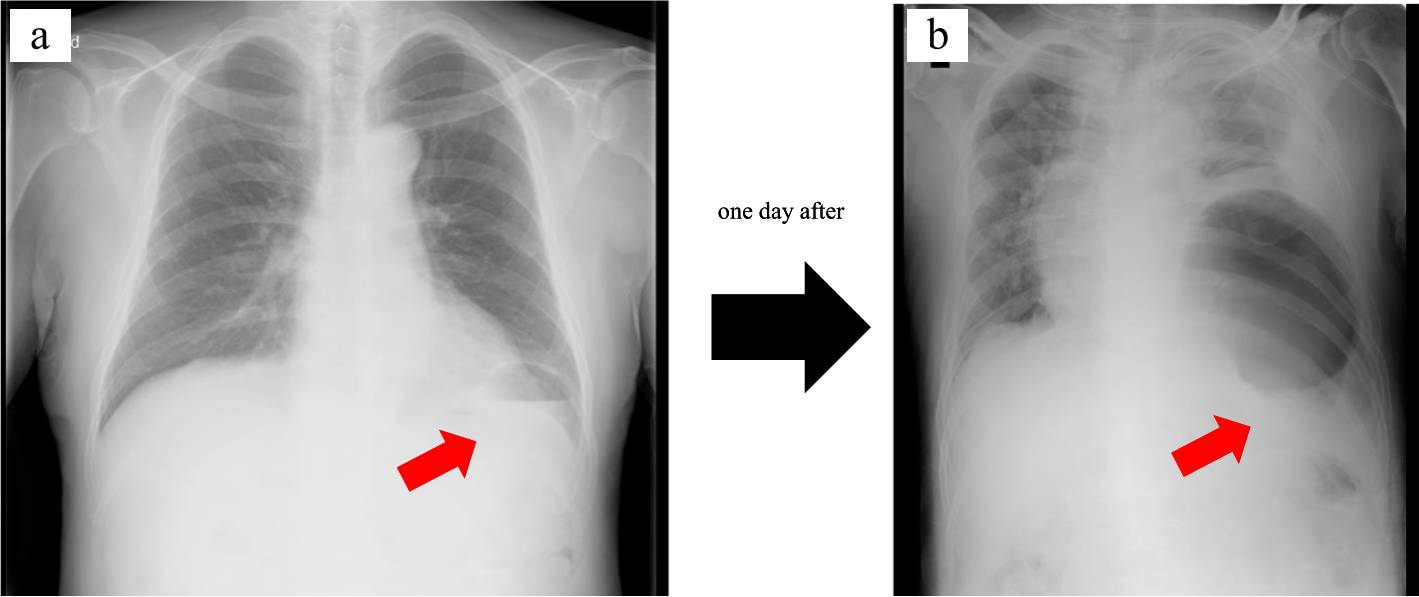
One day after hospitalization, chest X-ray showed acute exacerbation of the left diaphragmatic hernia (a, b)
